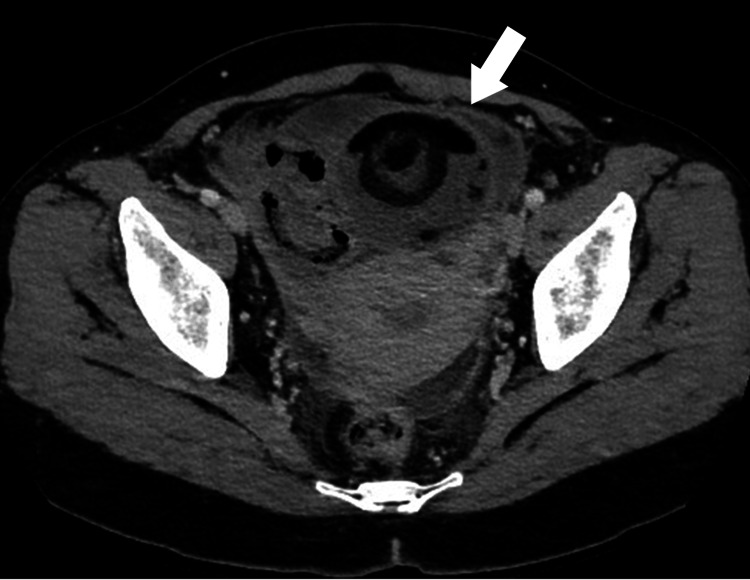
Abdominal-pelvic contrast-enhanced computed tomography showed a left ovarian tumor and ascites in the pelvis (arrow)
